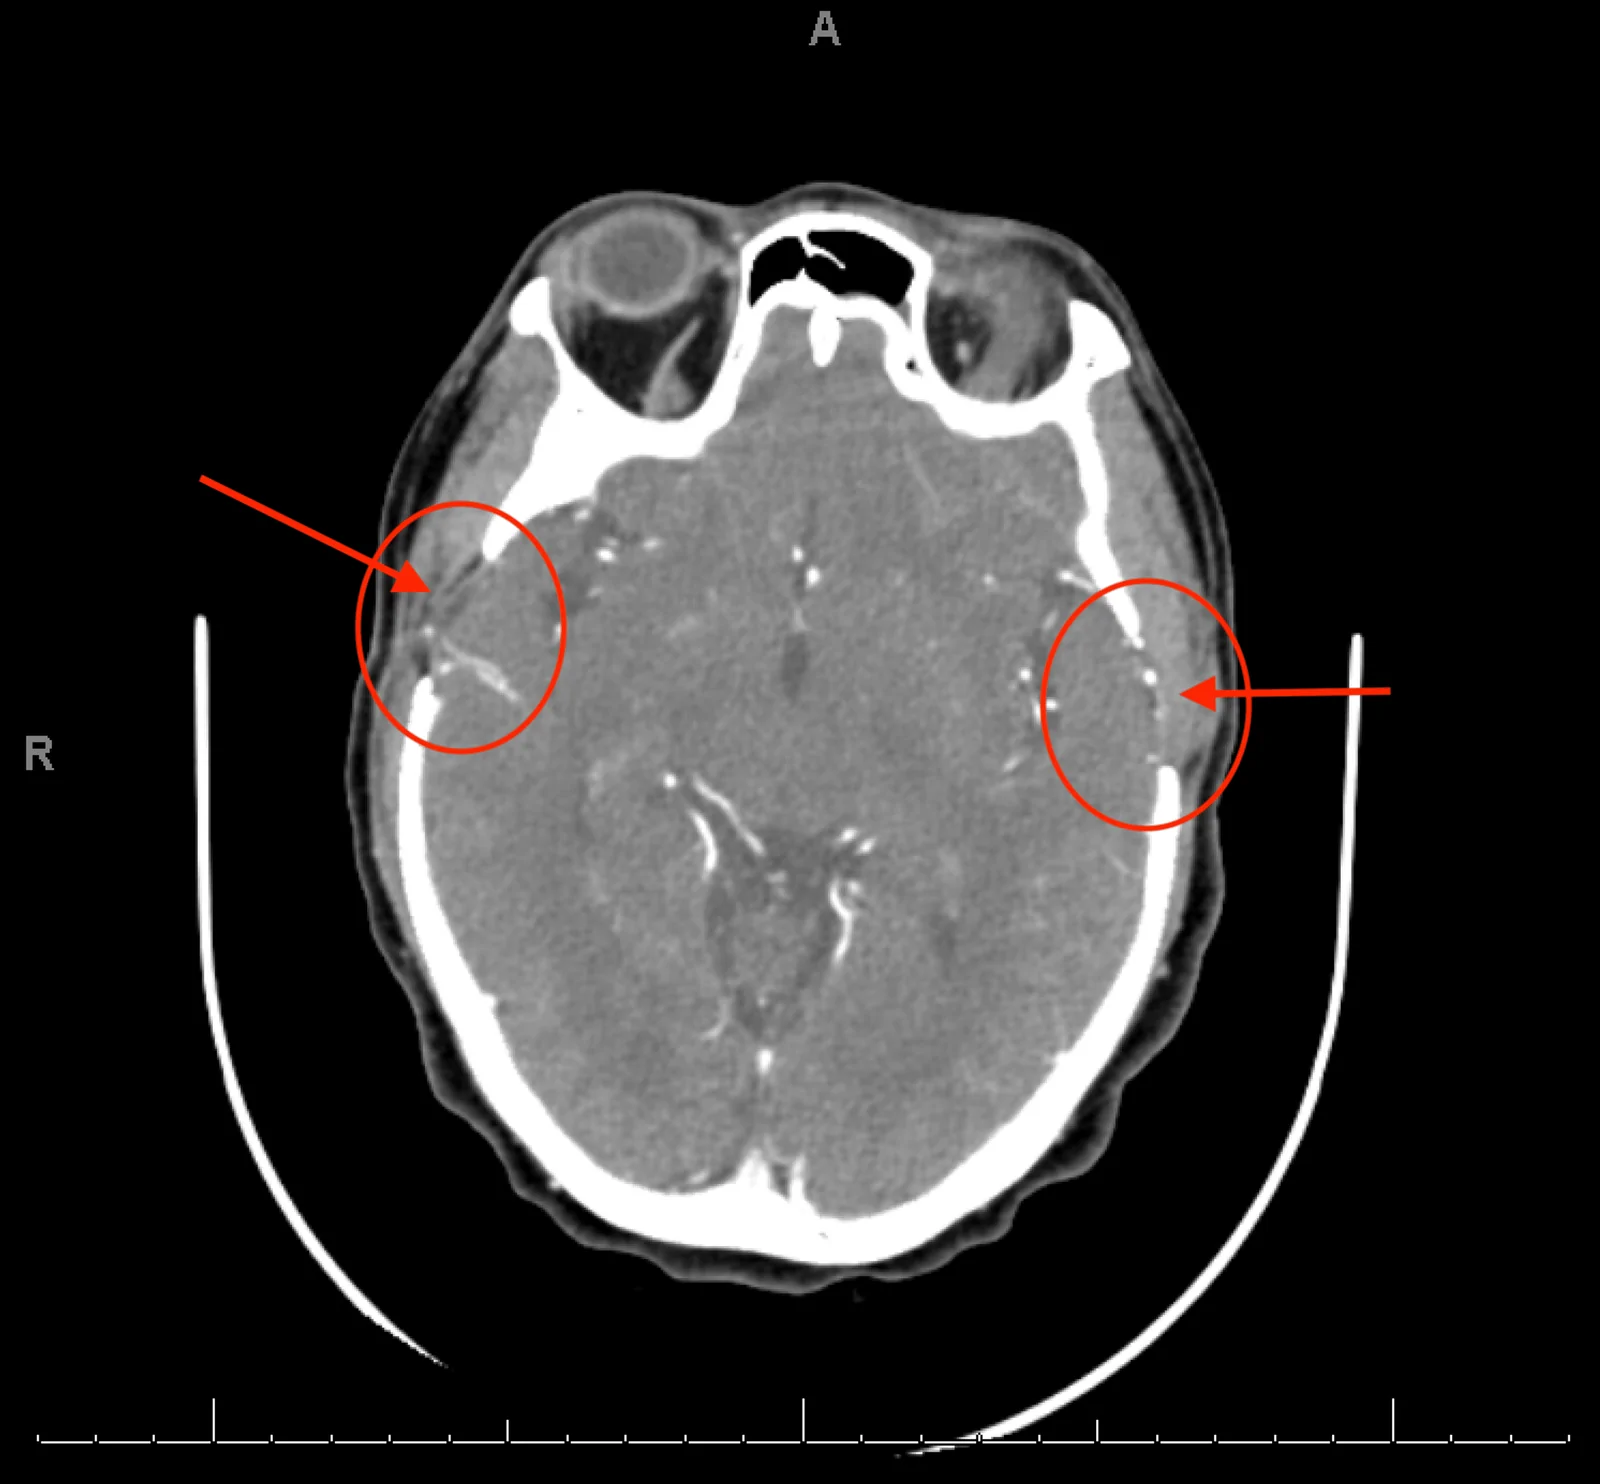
Noncontrast CT of the head demonstrating burr holes The circles in the image denote the locations of burr holes previously established in the patient during prior surgical interventions, performed as part of an indirect revascularization technique.
